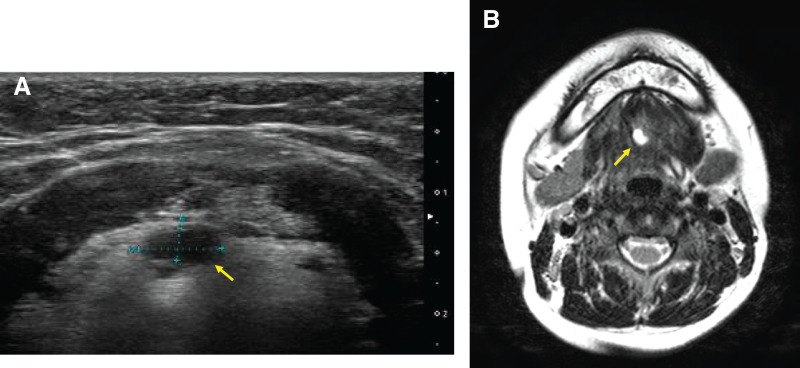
Preoperative findings. (A) Transcervical ultrasonography reveals a 7-mm cystic lesion (arrow) located on the dorsal side of the hyoid bone. (B) Neck MRI confirms the presence of a simple cyst (10 × 7 × 3 mm in size, arrow) near the midline between the hyoid bone and the foramen cecum.
